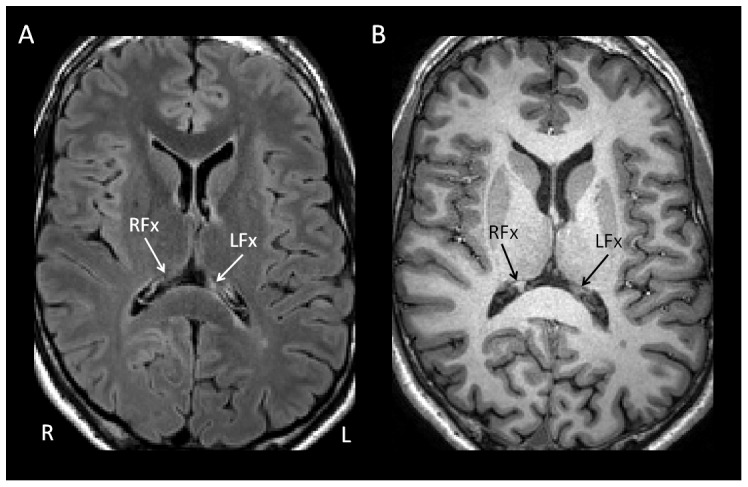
Identification of focal MS lesions of the fornix. (a) Axial FLAIR image from one of the participants with MS showing focal T2-hyperintesity in crus of the left fornix (LFx) with accompanying T1-hypointensity on the corresponding MPRAGE image (b). Crus of the right fornix labelled for comparison (RFx).
